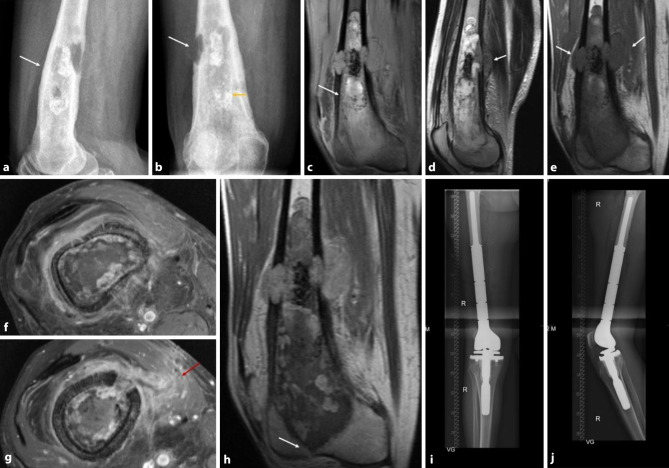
(Case 1). Anteroposterior (AP) and lateral radiographs of the distal femur showing an osteoblastic-osteolytic lesion (a, white arrows) with poorly defined margins and subtle periosteal reaction at the medial aspect of the distal femur. The lesion demonstrates a broad transition zone with a cartilaginous matrix (yellow arrow), cortical destruction and thinning (b). Coronal and sagittal T2-weighted MRI with and without fat saturation showing a heterogeneous osteodestructive lesion with typical nodular enhancement and significant mucoid component, surrounding bone marrow oedema (c) in the distal femur with cortical disruption (d). (e) Extraosseous tumor component along the bone circumference with low signal intensity. Postcontrast axial T1-weighted fat-saturated MRI sequences on two consecutive slices showing the extent of the lesion with circumferential cortical infiltration, heterogeneous contrast enhancement of the extraosseous tumor component in the prefemoral fat and biopsy tract on the medial side of the upper leg (red arrow) (f, g). The distal extension of the tumor without the signs of joint infiltration (h). Postoperative radiographs in AP and lateral after wide resection with built-in modular tumor endoprosthesis (i, j)
